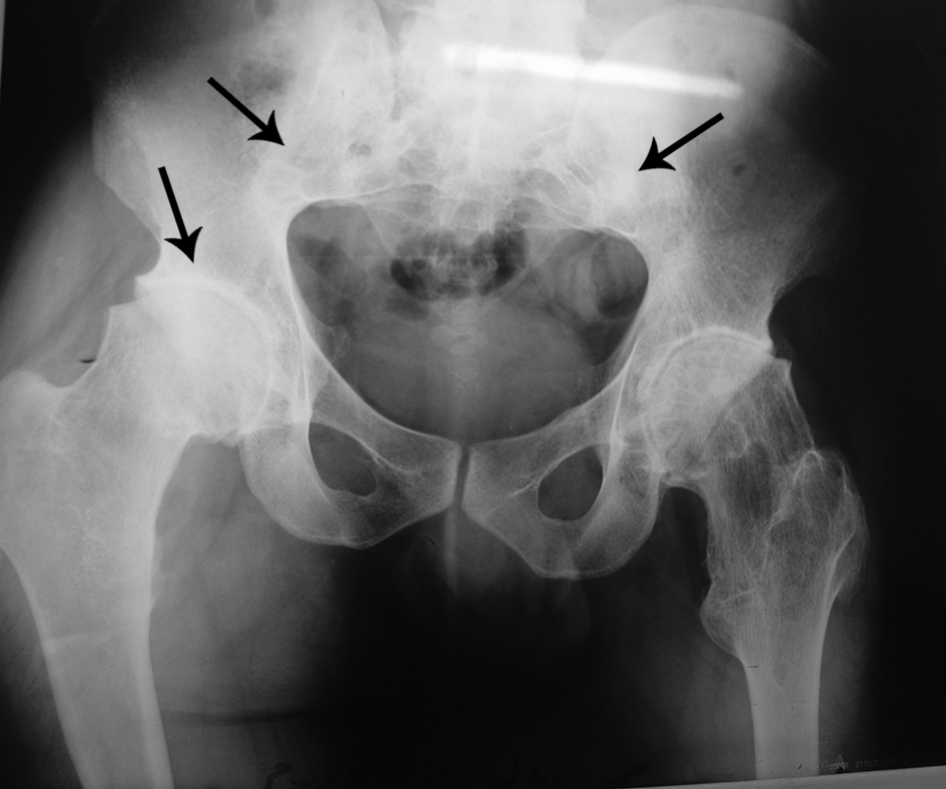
Reduction of the hip joint spaces and bilateral sacroiliitis. AP radiograph of the hip shows symmetrical narrowing of both hip joint spaces (black arrow), bilateral sacroiliac joint erosions and iliac side subchondral sclerosis (bilateral sacroiliitis, the arrows at the top).
